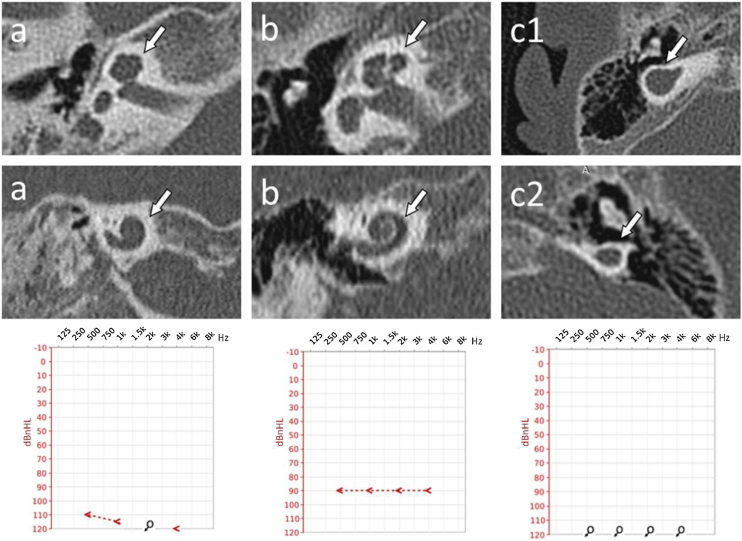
Cochlear malformations identified in computed tomography evaluation and presented in the planes selected for the most precise visualization. Each panel represents a particular patient: (a) Case #2 right ear, cochlear hypoplasia type II; (b) Case #1 right ear, cochlear hypoplasia type III; (c) Case #10 right ear, (c1) Cochlear Aplasia with a Dilated Vestibule (CADV); (c2) Rudimentary otocyst. White arrows indicate anomaly location. Individual cases are supplemented with ASSR reconstruction of audiograms (hearing thresholds in dBnHL), respectively.
